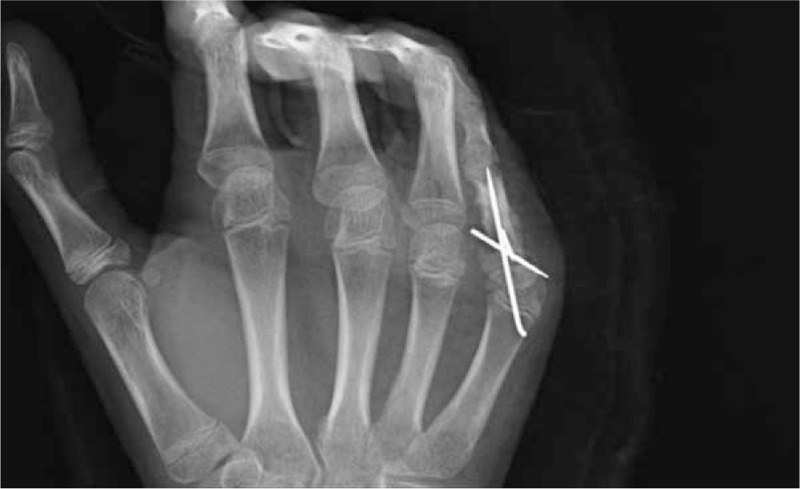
Postoperative oblique radiograph shows the correct alignment of the digit and 3 K-wires stabilize the fracture.
